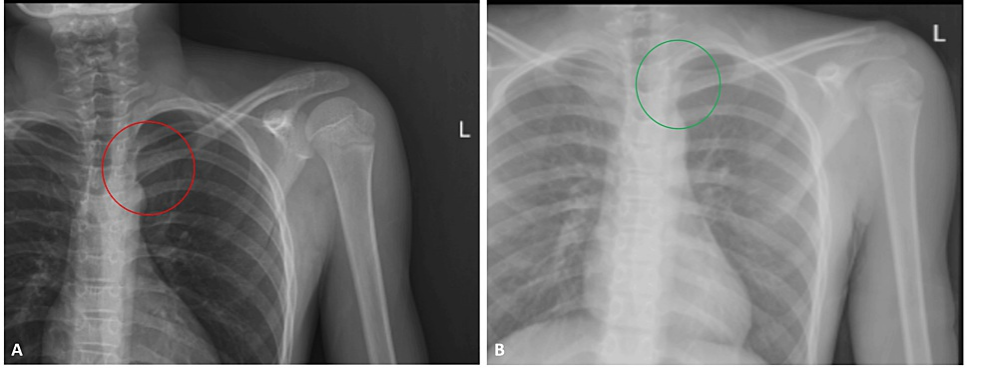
Left shoulder anteroposterior radiographs before the and after the reduction (A) Left shoulder anteroposterior radiograph before the reduction. The left sternoclavicular joint is marked with a red circle; (B) Left shoulder anteroposterior radiograph after the reduction. The left sternoclavicular joint is marked with a green circle.
